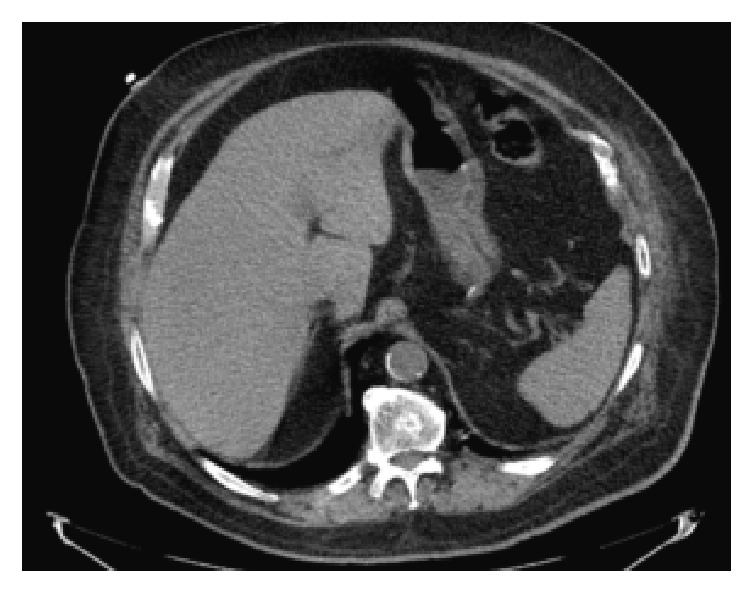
CT of abdomen with contrast showing unremarkable liver, spleen, pancreas, and adrenal glands.
